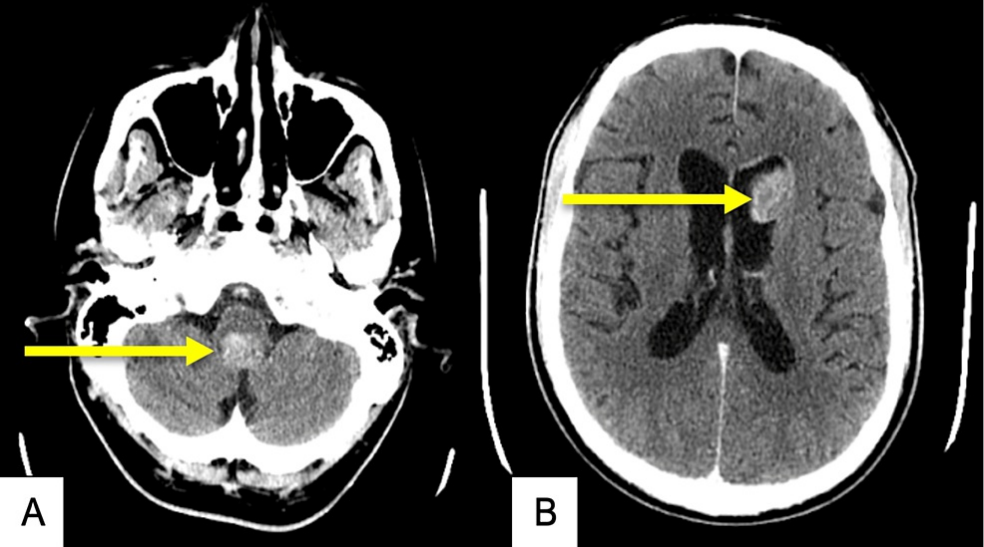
CT scan of the brain, axial view (A-B) Contrast scans showing two enhancing soft tissue masses (yellow arrows) in the base of the fourth ventricle and anterior horn of the left lateral ventricle
